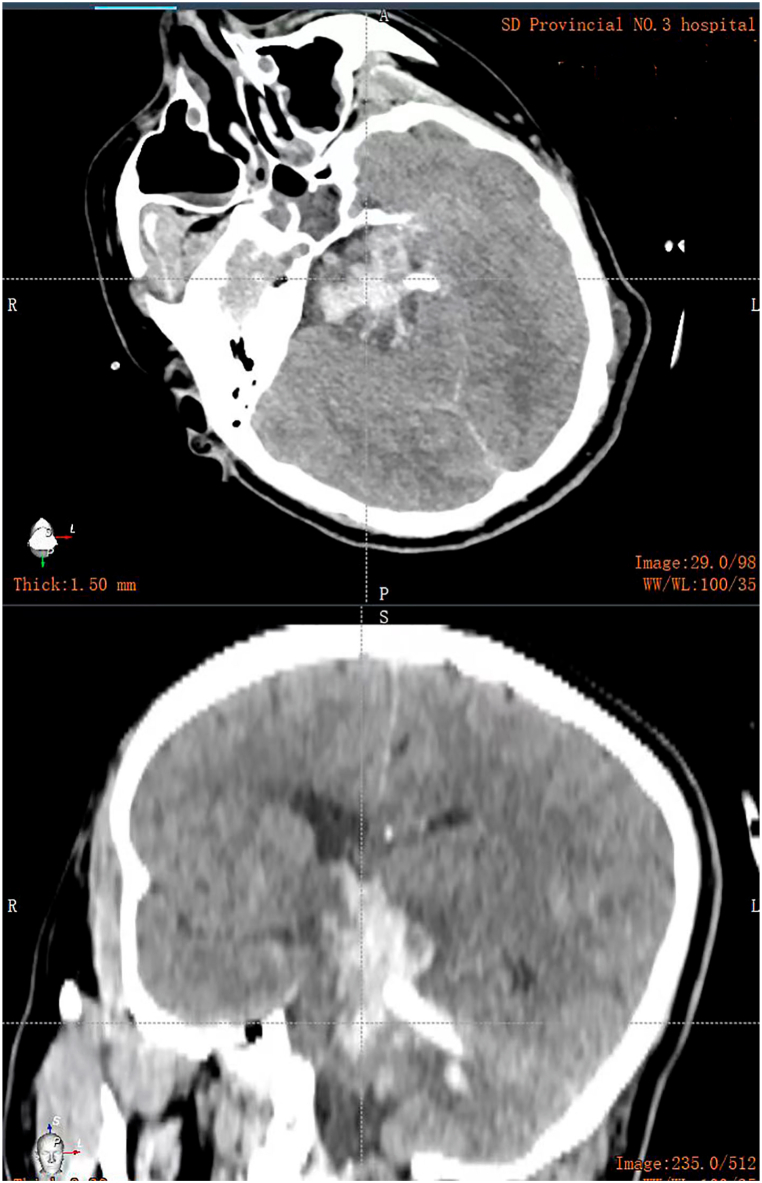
Immediate postoperative CT of case 3.
